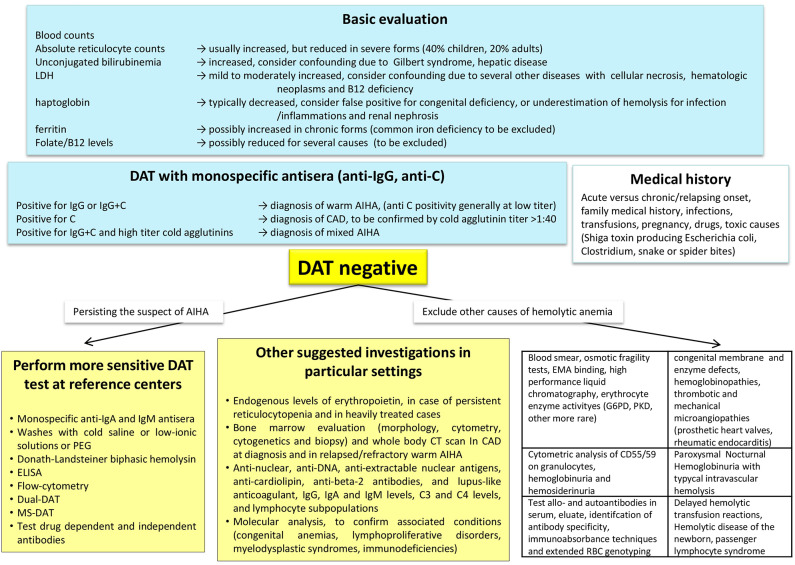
Diagnostic algorithm of autoimmune haemolytic anemia (AIHA). DAT, direct antiglobulin test or Coombs test; MS-DAT, mitogen-stimulated DAT; CAD, cold agglutinin disease; CT, computed tomography; G6PD, glucose-6-phosphate dehydrogenase deficiency; PKD, pyruvate kinase deficiency; EMA-binding, eosin-5′-maleimide-binding test.
